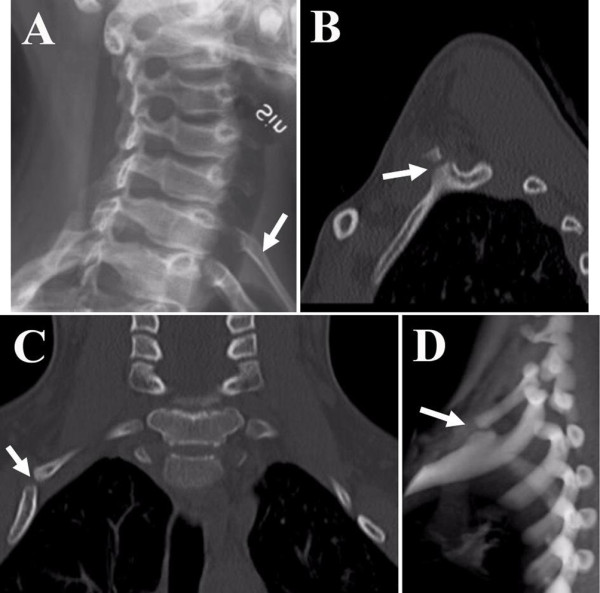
Case 1: (A) Plain radiograph oblique view showing the right cervical rib (arrow). (B-D) CT sagittal, coronal and 3D-reconstructed images showing the pseudoarthrotic bony formation (arrows) between the cervical rib and the first rib.
